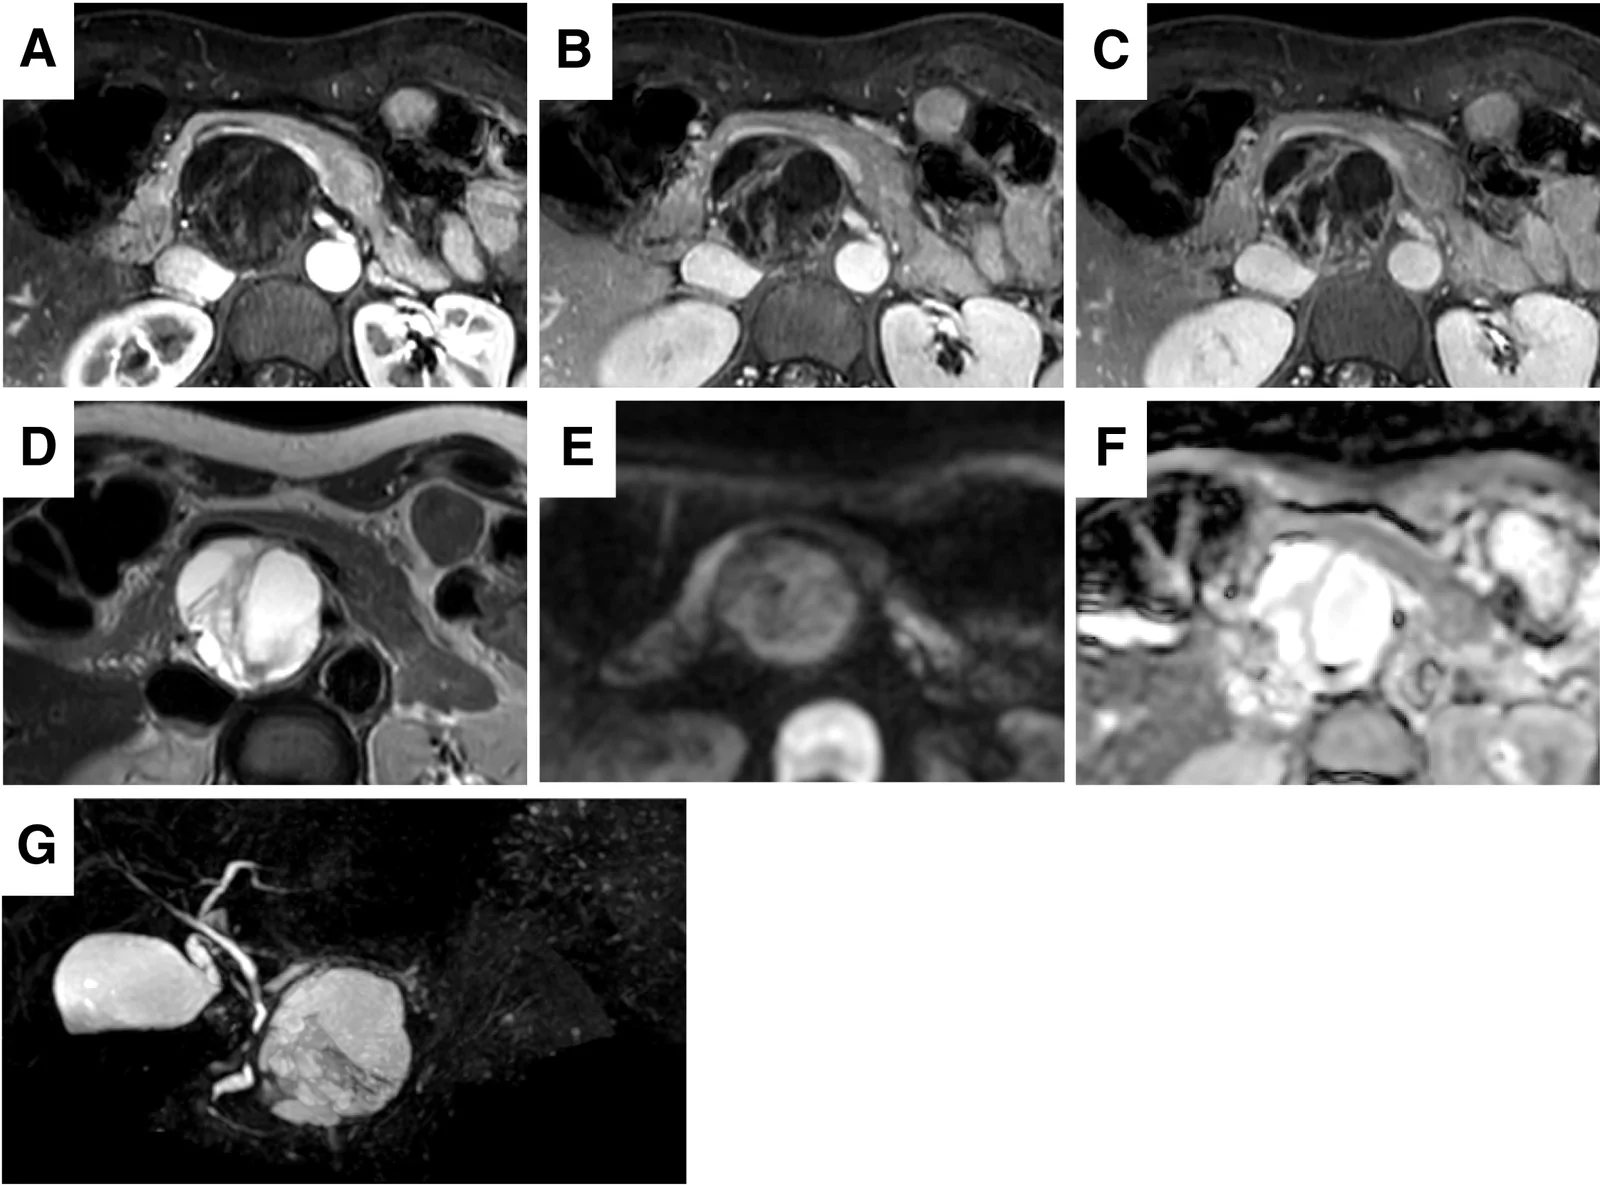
MRI and MRCP findings. (A–C) Dynamic contrast-enhanced, fat-suppressed T1-weighted images obtained during the arterial (A), portal venous (B), and equilibrium (C) phases showing gradual enhancement of the septa and inner cyst wall. (D) T2-weighted image showing a multilocular cystic lesion dorsal to the pancreatic head, with locules of various sizes separated by irregular septa. (E) Diffusion-weighted image showing relatively high signal intensity in the thickened septal portions. (F) ADC map showing no definite diffusion restriction throughout the lesion. (G) MRCP showing no obvious communication between the lesion and the main pancreatic duct. ADC, apparent diffusion coefficient; MRCP, magnetic resonance cholangiopancreatography
